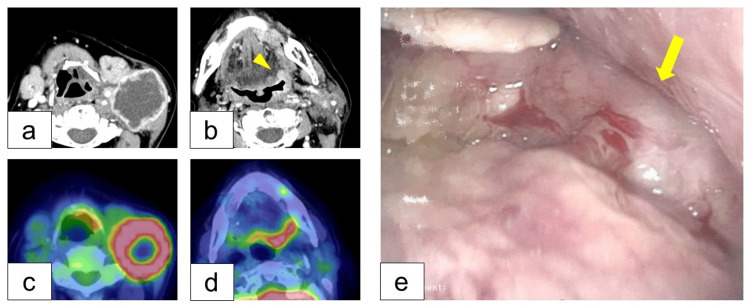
Cervical Lymph Node Metastasis and Mesopharyngeal Tumor Contrast-enhanced computed tomography (CT) showed a 5 cm tumor with ring enhancement in the left cervical lymph node (a) and the left oropharynx (b), both exhibiting increased fluorodeoxyglucose (FDG) uptake on positron emission tomography CT (c, d). (e) Pharyngoscopy revealed a hypervascular lesion with whitish exudate on the left wall of the oropharynx (arrow).
